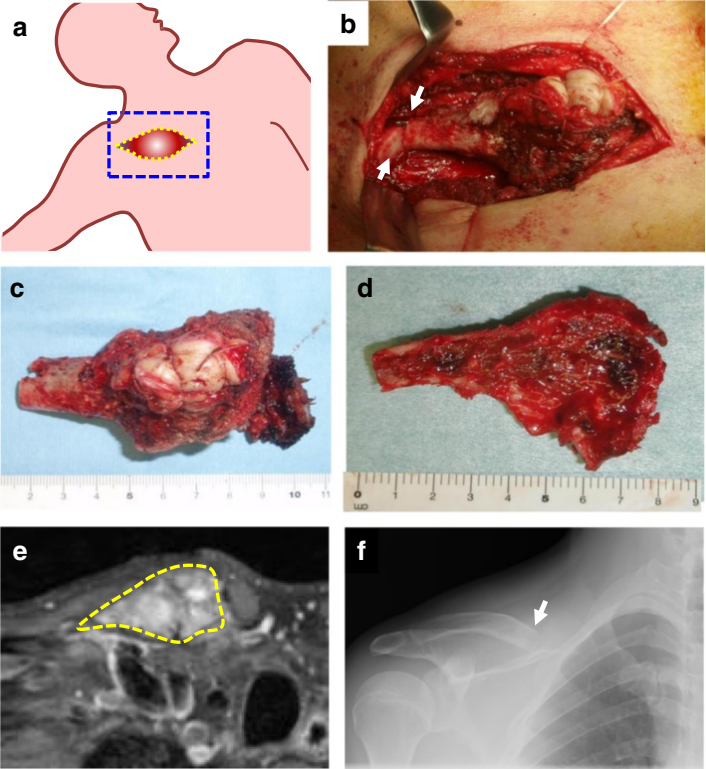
Intraoperative findings. (a) In the supine position with the right arm abducted, the right clavicle was carefully exposed without damaging the tumor capsule. (b) An osteotomy was made 8 cm from the proximal edge of the clavicle (*arrows*). (c) Disarticulation of the sternoclavicular joint was then performed. (d) The tumor, resected en-bloc with half of the clavicle, had a thin, fragile cortex, but no extension to the soft tissue. (e) The residual clavicular length was 7.5 cm on postoperative roentgenography. (f) No displacement of the resection edge of the clavicle was observed
